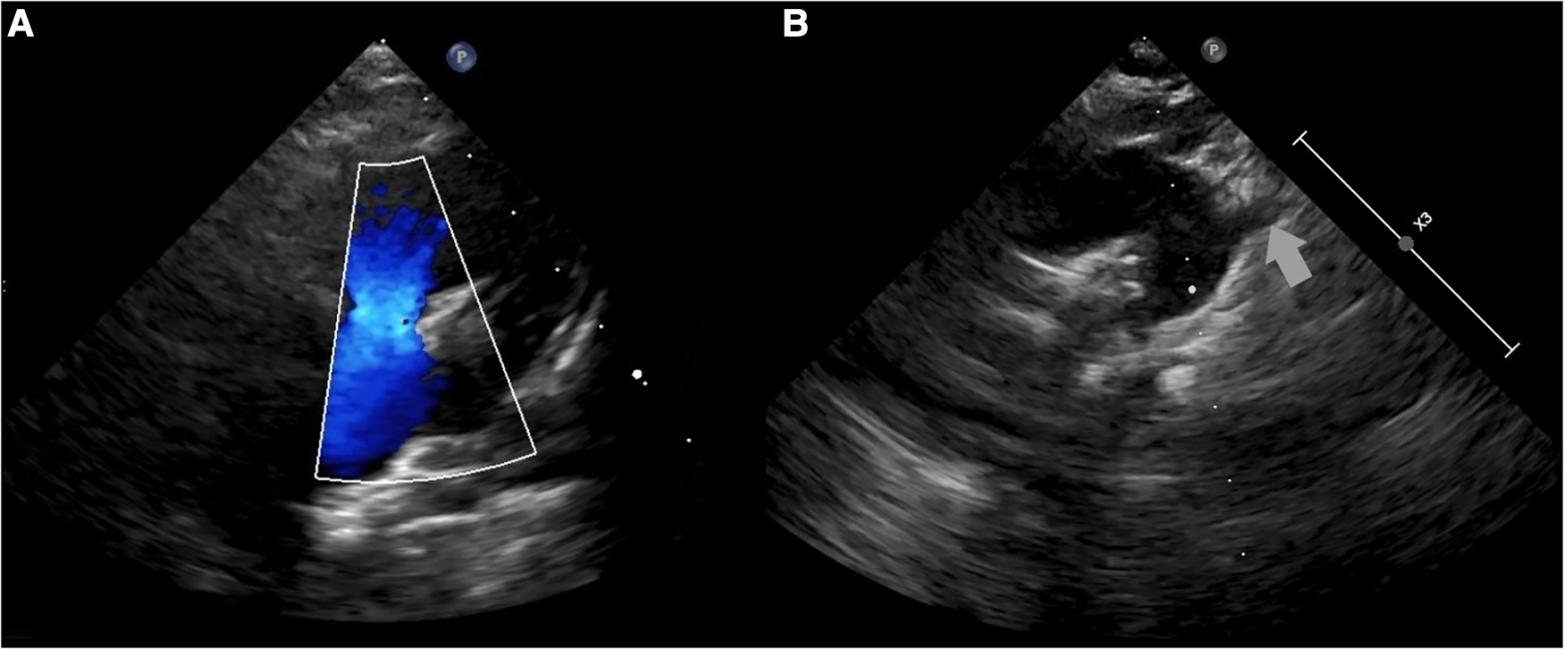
A transthoracic echocardiogram: (A) color Doppler flow not registered in the left pulmonary artery; (B) suspected right arch of aorta and anomaly of subclavian arteries.
